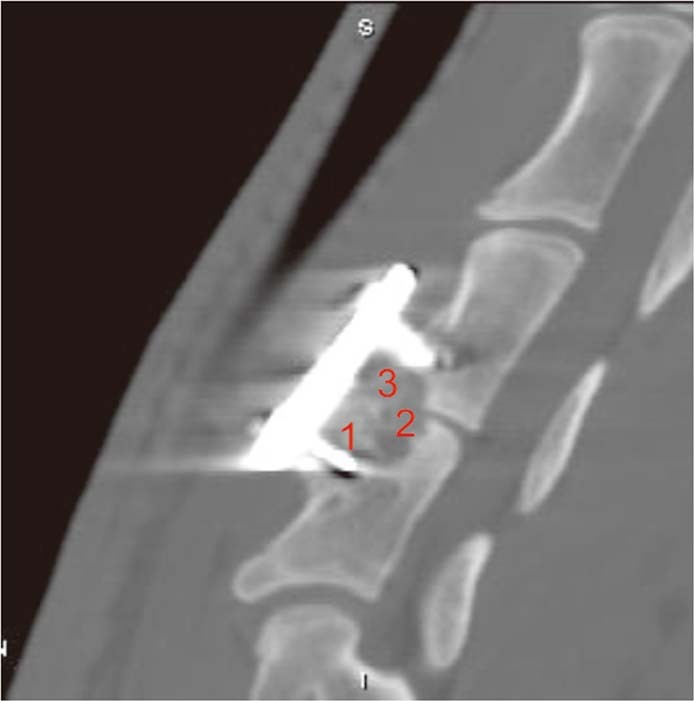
Radiographic assessment. Four sagittal planes at the C3 and C4 segments were reconstructed with 2-mm slice thickness. The interface between the implant and vertical bone on each plane was divided into 1, 2 and 3 zones for the degree of fusion status. The scoring criteria were described by van Dijk and graded as follows: 0= pseudoarthrosis; 1= ingrowth of bone with the strut securely fixed to vertebral bone above and below, but with a radiolucent discontinuity in the fusion mass; 2= arthrodesis with solid bone bridging the fusion area. The total points for each sample was 24 points.
